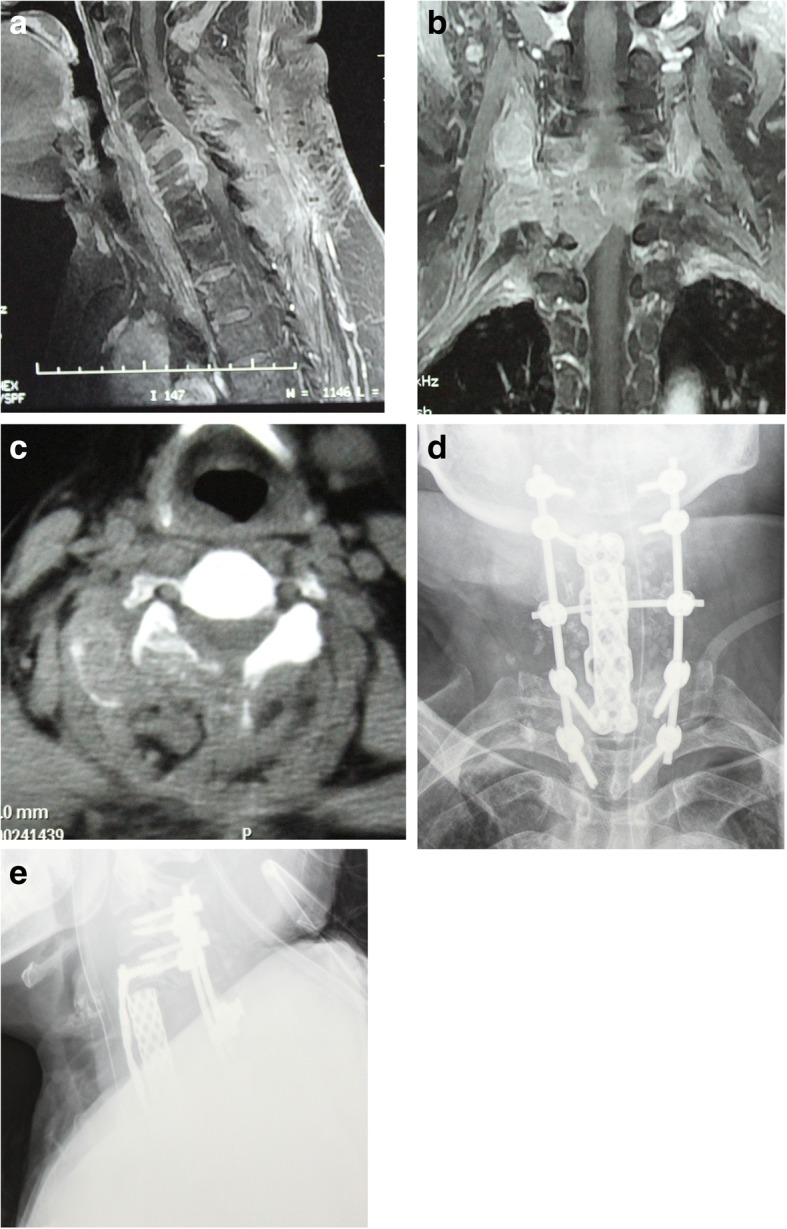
Patient no. 14: MRI showing a vertebral lesion with a paraspinal mass from C5 to C7 (a, b). CT images (c) showing an osteolytic lesion with calcification. AP (d) and LAT (e) X-rays after surgery, showing anterior vertebral reconstruction and posterior internal fixation
